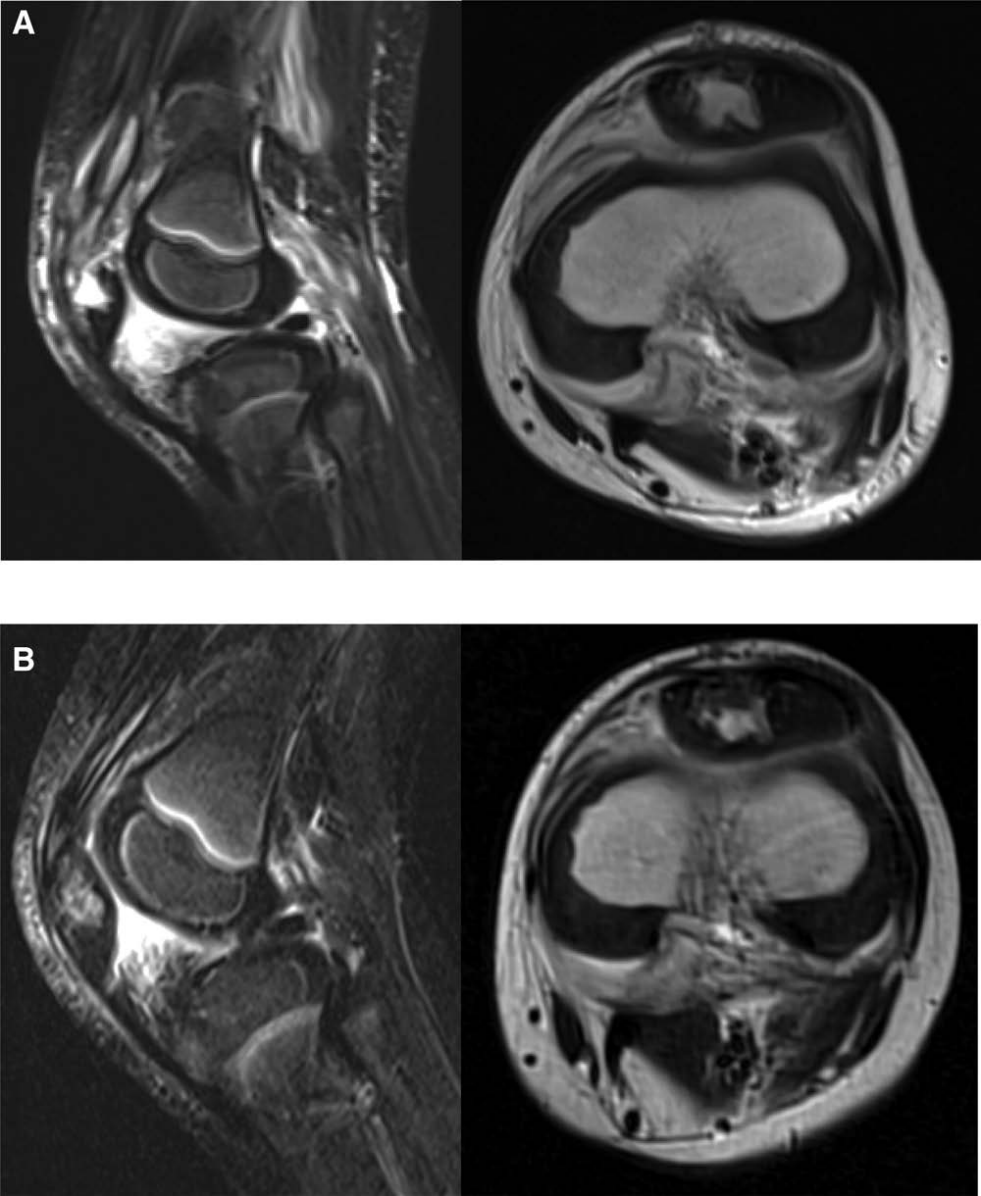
A repeat magnetic resonance imaging (MRI) of the left knee obtained after the referral to our institution. Short-tau inversion recovery (STIR) sagittal (left) and T2-weighted axial (right) MRI images obtained 19 days (A) and 39 days (B) after discontinuation of continuous intracapsular irrigation. MRI = magnetic resonance imaging.
